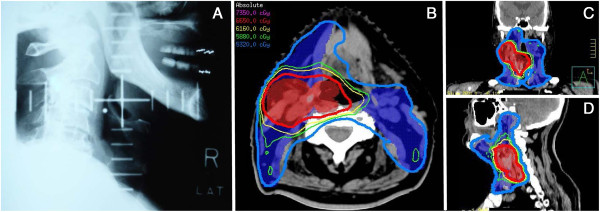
Evolution of radiotherapy planning. Historically, radiation treatment planning was based on bony landmarks as seen using 2-dimensional x-ray films (A). Modern radiotherapy allows for sculpting of radiotherapy dose in three dimensions (axial B; coronal C; sagittal D), with differential doses to high-risk (thick red line) and low-risk (thick blue line) areas, and relative sparing of the normal tissues including parotid glands, spinal cord and brain.
